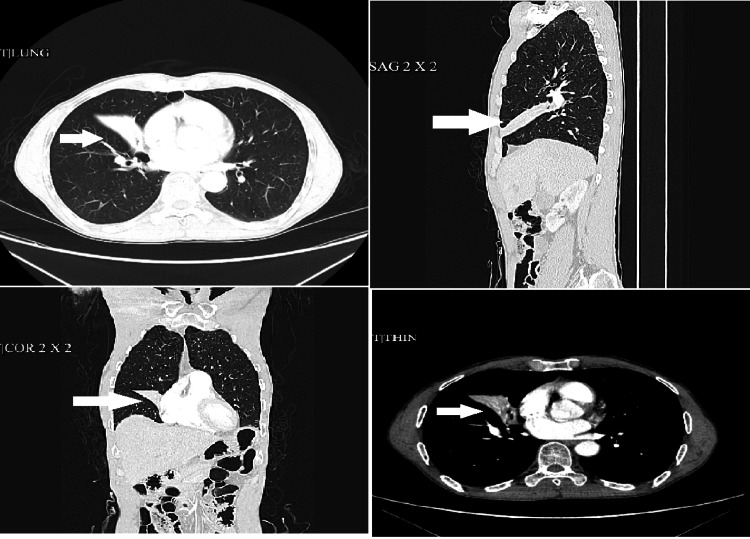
CT scan of the lung, total collapse of the right middle lobe
